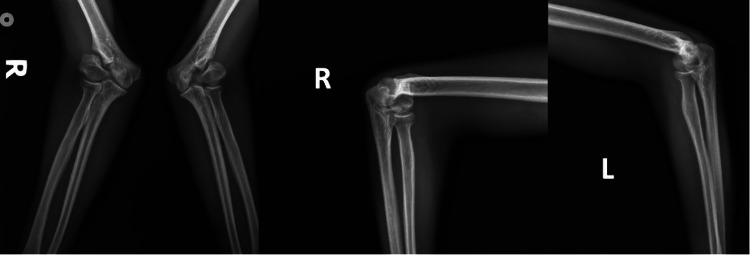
Radiographic evaluation revealed non-union in both elbows caused by previous LHC fractures, accompanied by pathological valgus deformities LHC: lateral humeral condyle
